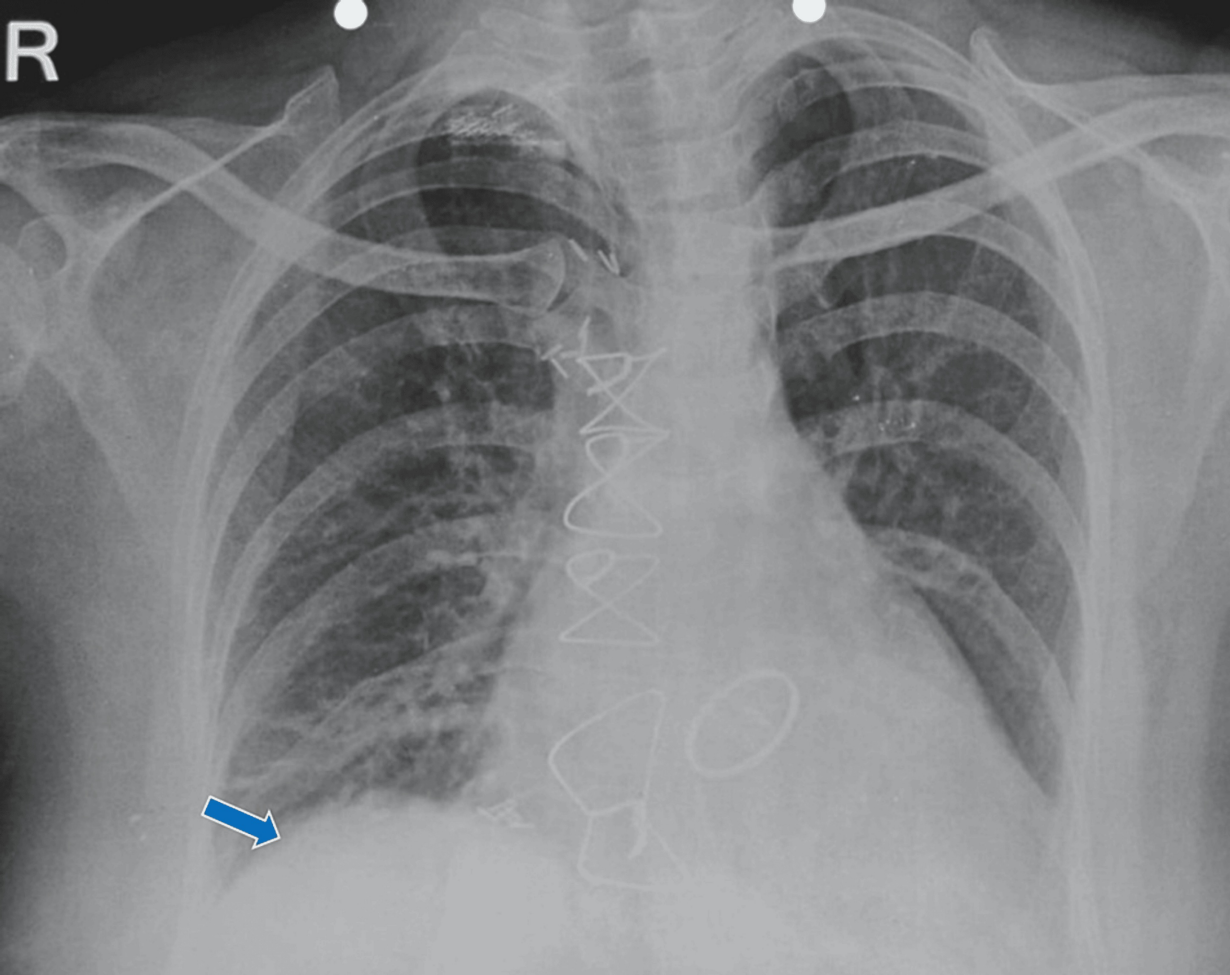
Chest radiograph showing near-complete resolution of the lesion (blue arrow) a month after diaphragmatic plication.
